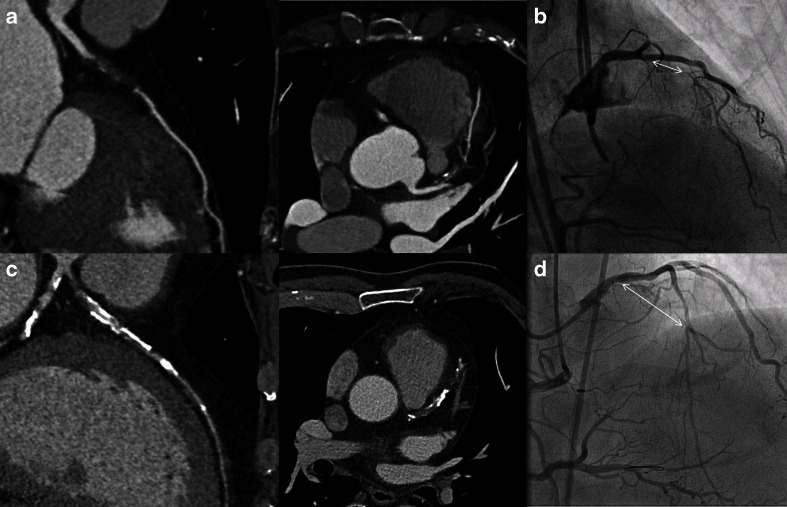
CTO wire crossing difficulty score appreciated from CCTA and ICA. The *top* CCTA (a) and ICA (b) images display a short occlusion (<20 mm) (*white arrow*), tapered proximal stump, no calcification, and no bending of the occluded segment (low CT-RECTOR score). This occlusion should be approached and probably successfully crossed with antegrade wire escalation technique. The *bottom* CCTA (c) and ICA (d) images clearly demonstrates a long (>20 mm) (*white arrow*) calcified occlusion (high CT-RECTOR score) suggesting that the operator should be able to use dissection and re-entry techniques before trying to cross this occlusion because anatomy dictates which approach should be used. Abbreviations as in Figs. 2 and 3
